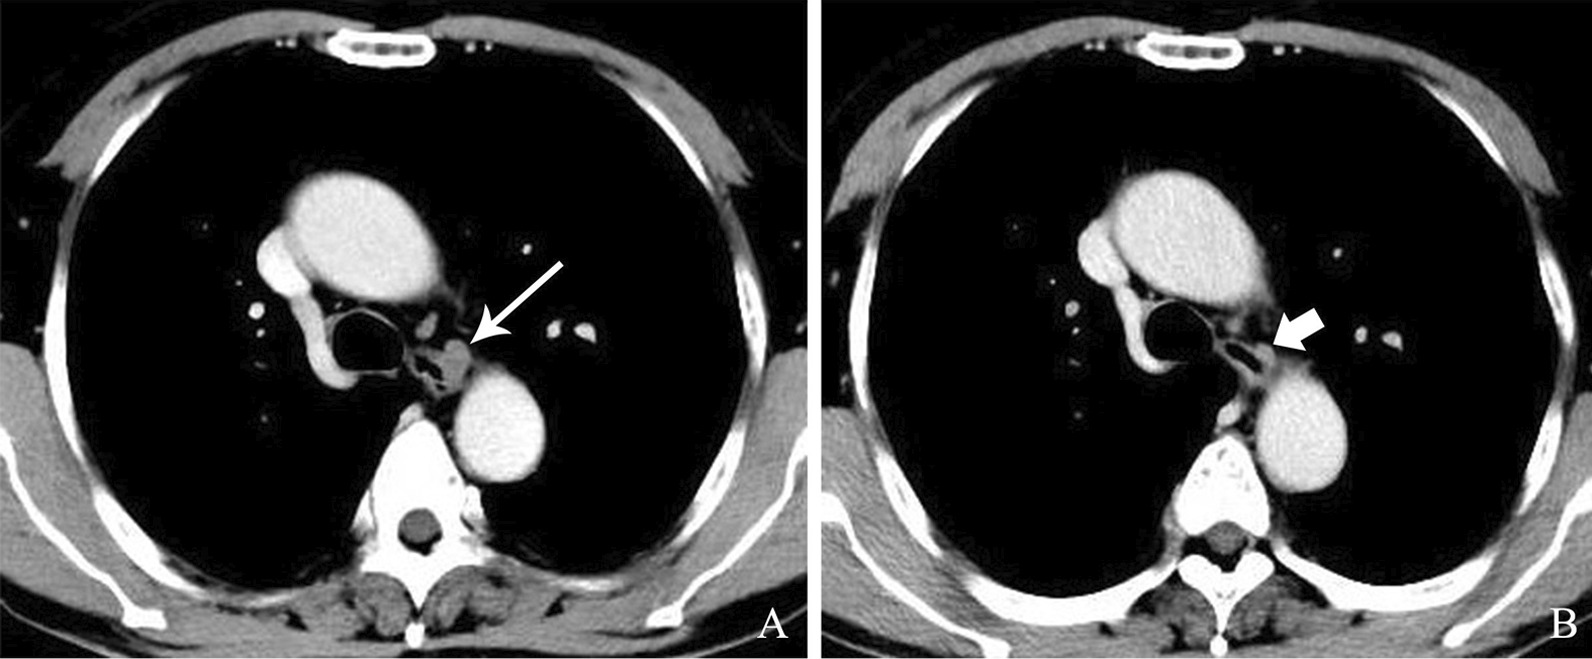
Contrast enhancement CT scan before and after anti-tuberculosis treatment. A Contrast enhancement CT scan before ATT showed a homogenous density mass (long arrow) adjacent to esophageal wall. B After ATT, follow-up contrast enhancement CT scan showed an obviously shrunk mass (short arrow)
